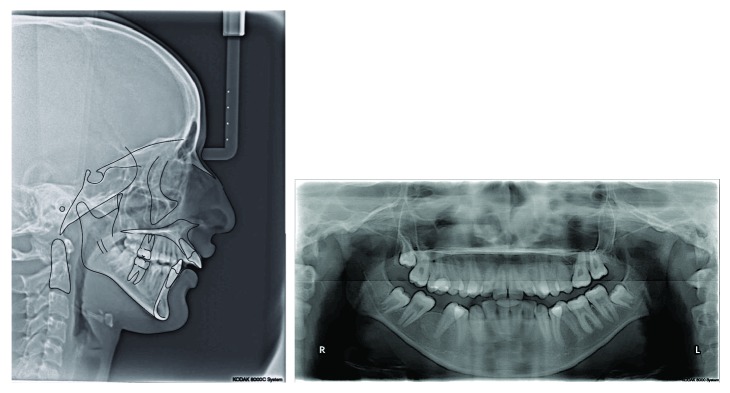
Pretreatment cephalometric and panoramic radiograph.
